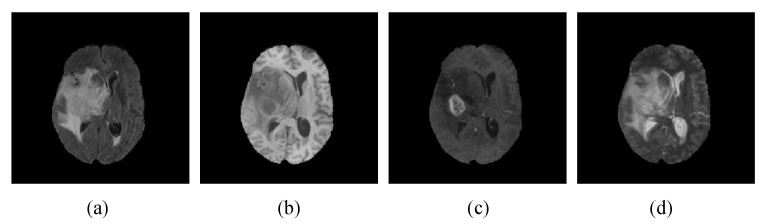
MRI images in different modalities: (a) Flair, (b) T1, (c) T1ce, and (d) T2.
